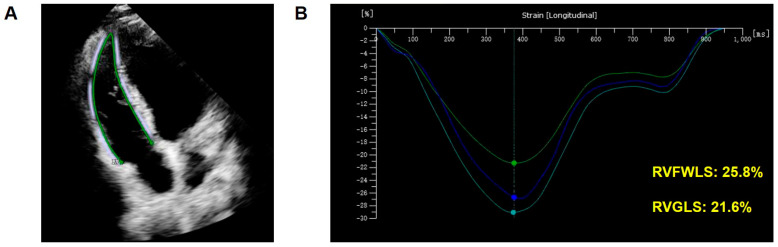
Example of assessment of RV systolic function by 2D-STE in a normal healthy person. (A) Tracing of 2D-RV longitudinal strain. (B) Processing of 2D-RV longitudinal strain. The green line represents the longitudinal strain of apical-free wall. The dark blue line represents the longitudinal strain of basal-free wall. The light blue line represents the longitudinal strain of mid-free wall. RV: right ventricular; 2D: two-dimensional; STE: speckle-tracking echocardiography.
